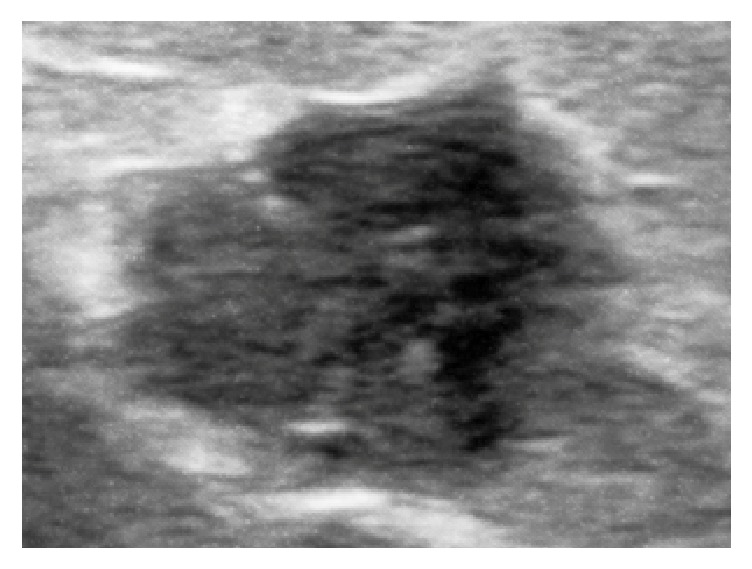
Ultrasound examination of the axillary mass reveals a hypoechoic mass with irregular margins.
